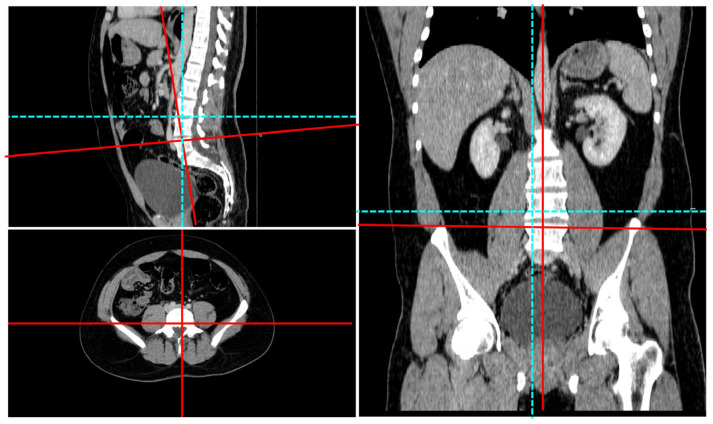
Multiplanar display of the CT image and adjustment of the planes according to the orientation of the superior endplate of the L5 vertebral body. The blue lines indicate the standard planes, while the red lines represent the adjusted planes.
